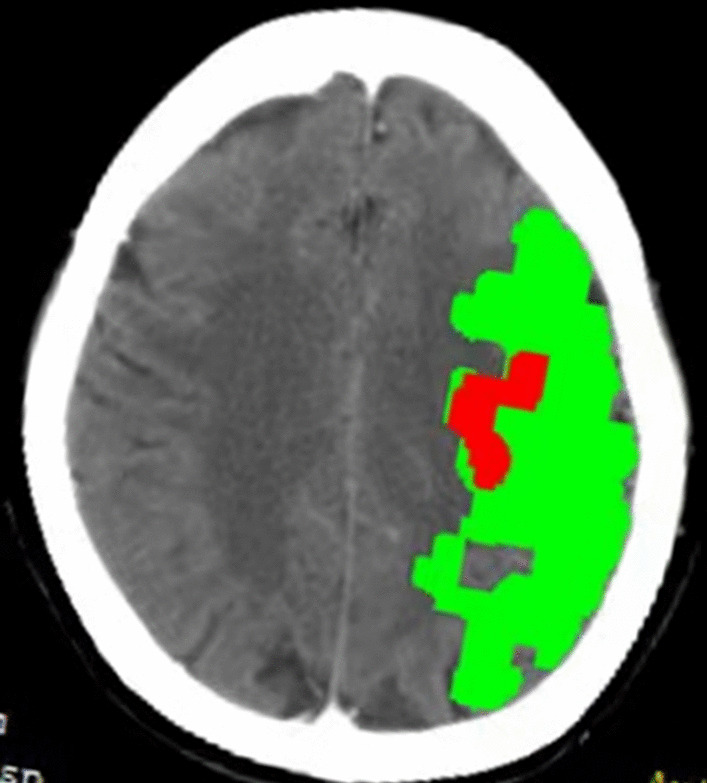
CT perfusion imaging images reconstructed by post-processing software MISta. CTP infarct core volume (Red): CBF＜30%. CTP ischemic penumbra volume (Green):Mismatch. CTP ischemic volume (Red plus Green): DT>3s^+^
